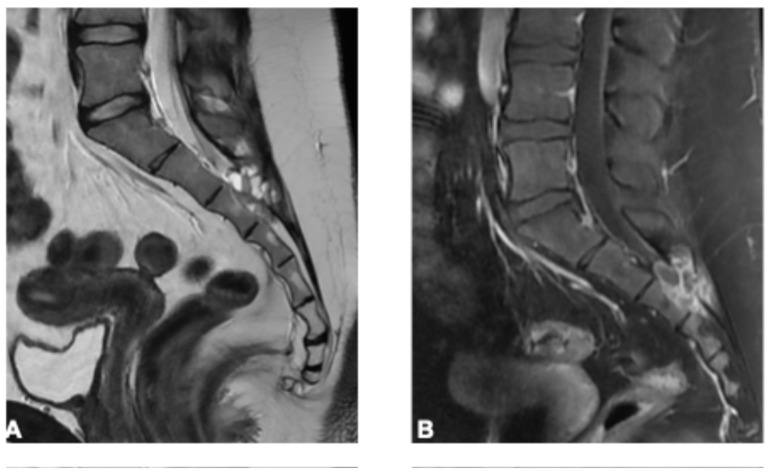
Aneurysmal bone cyst in a 12-year-old girl. Sagittal T2-weighted MRI (A) shows an expansile, multiloculated cystic lesion involving the posterior elements of the sacrum and extending into the spinal canal. The lesion demonstrates multiple fluid–fluid levels, consistent with hemorrhagic content. Post-contrast fat-suppressed T1-weighted image (B) reveals peripheral and septal enhancement.
